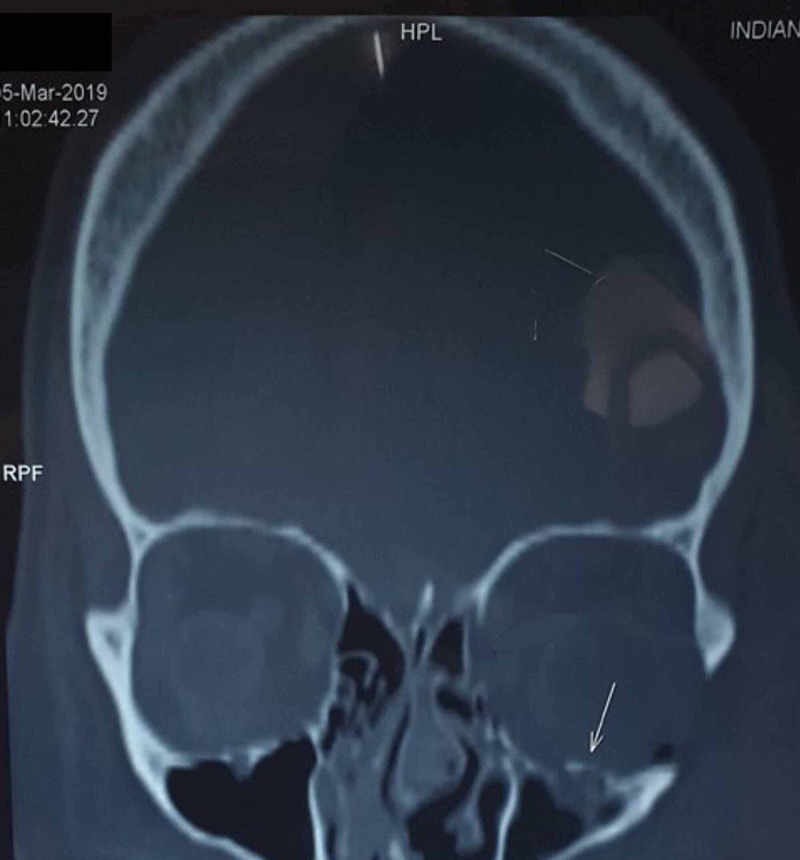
Preop CT showing left orbital floor fracture
